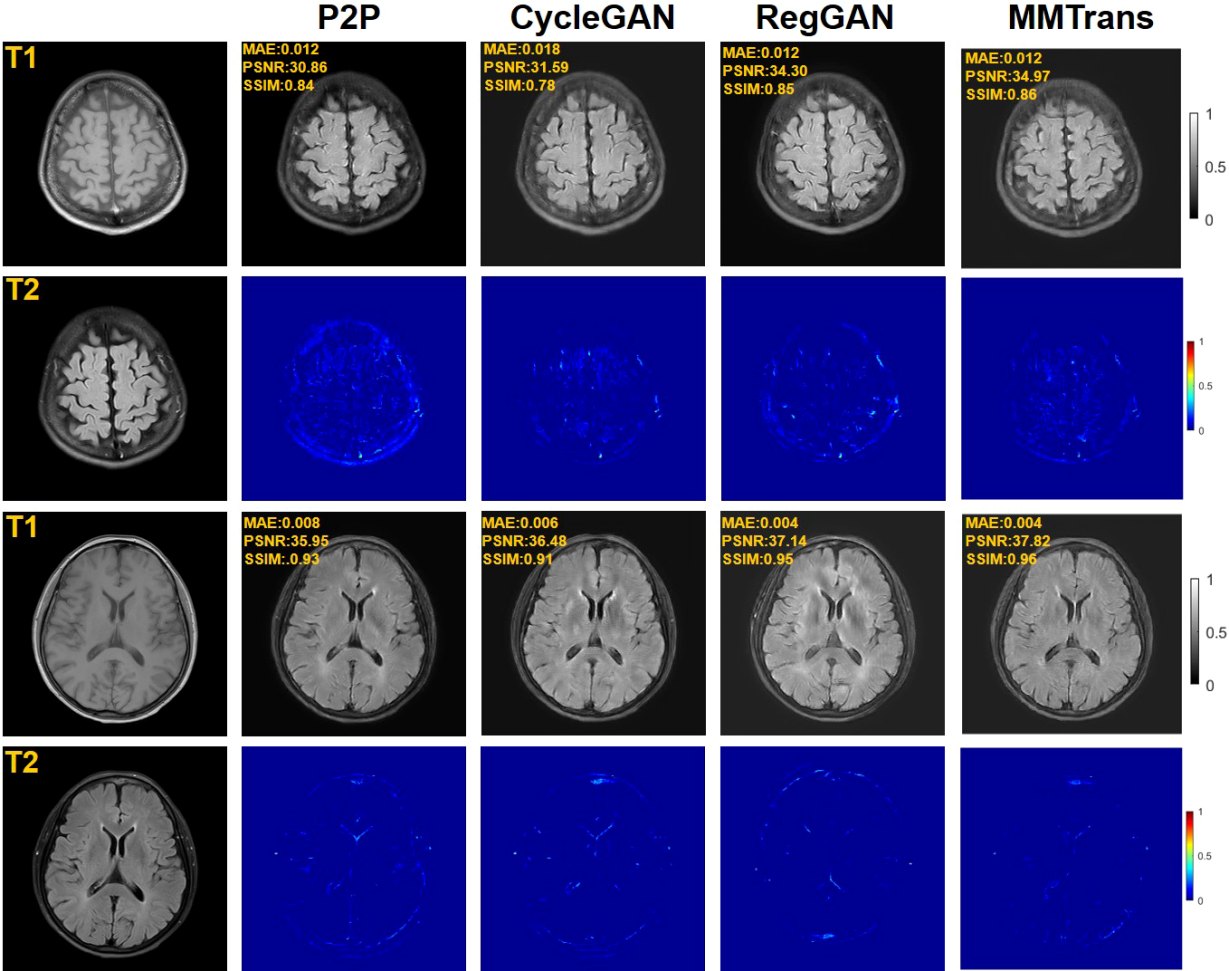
Qualitative results of different translation methods for translating T2 from T1 using the paired clinical brain MRI dataset, showing translation images and corresponding error maps.
